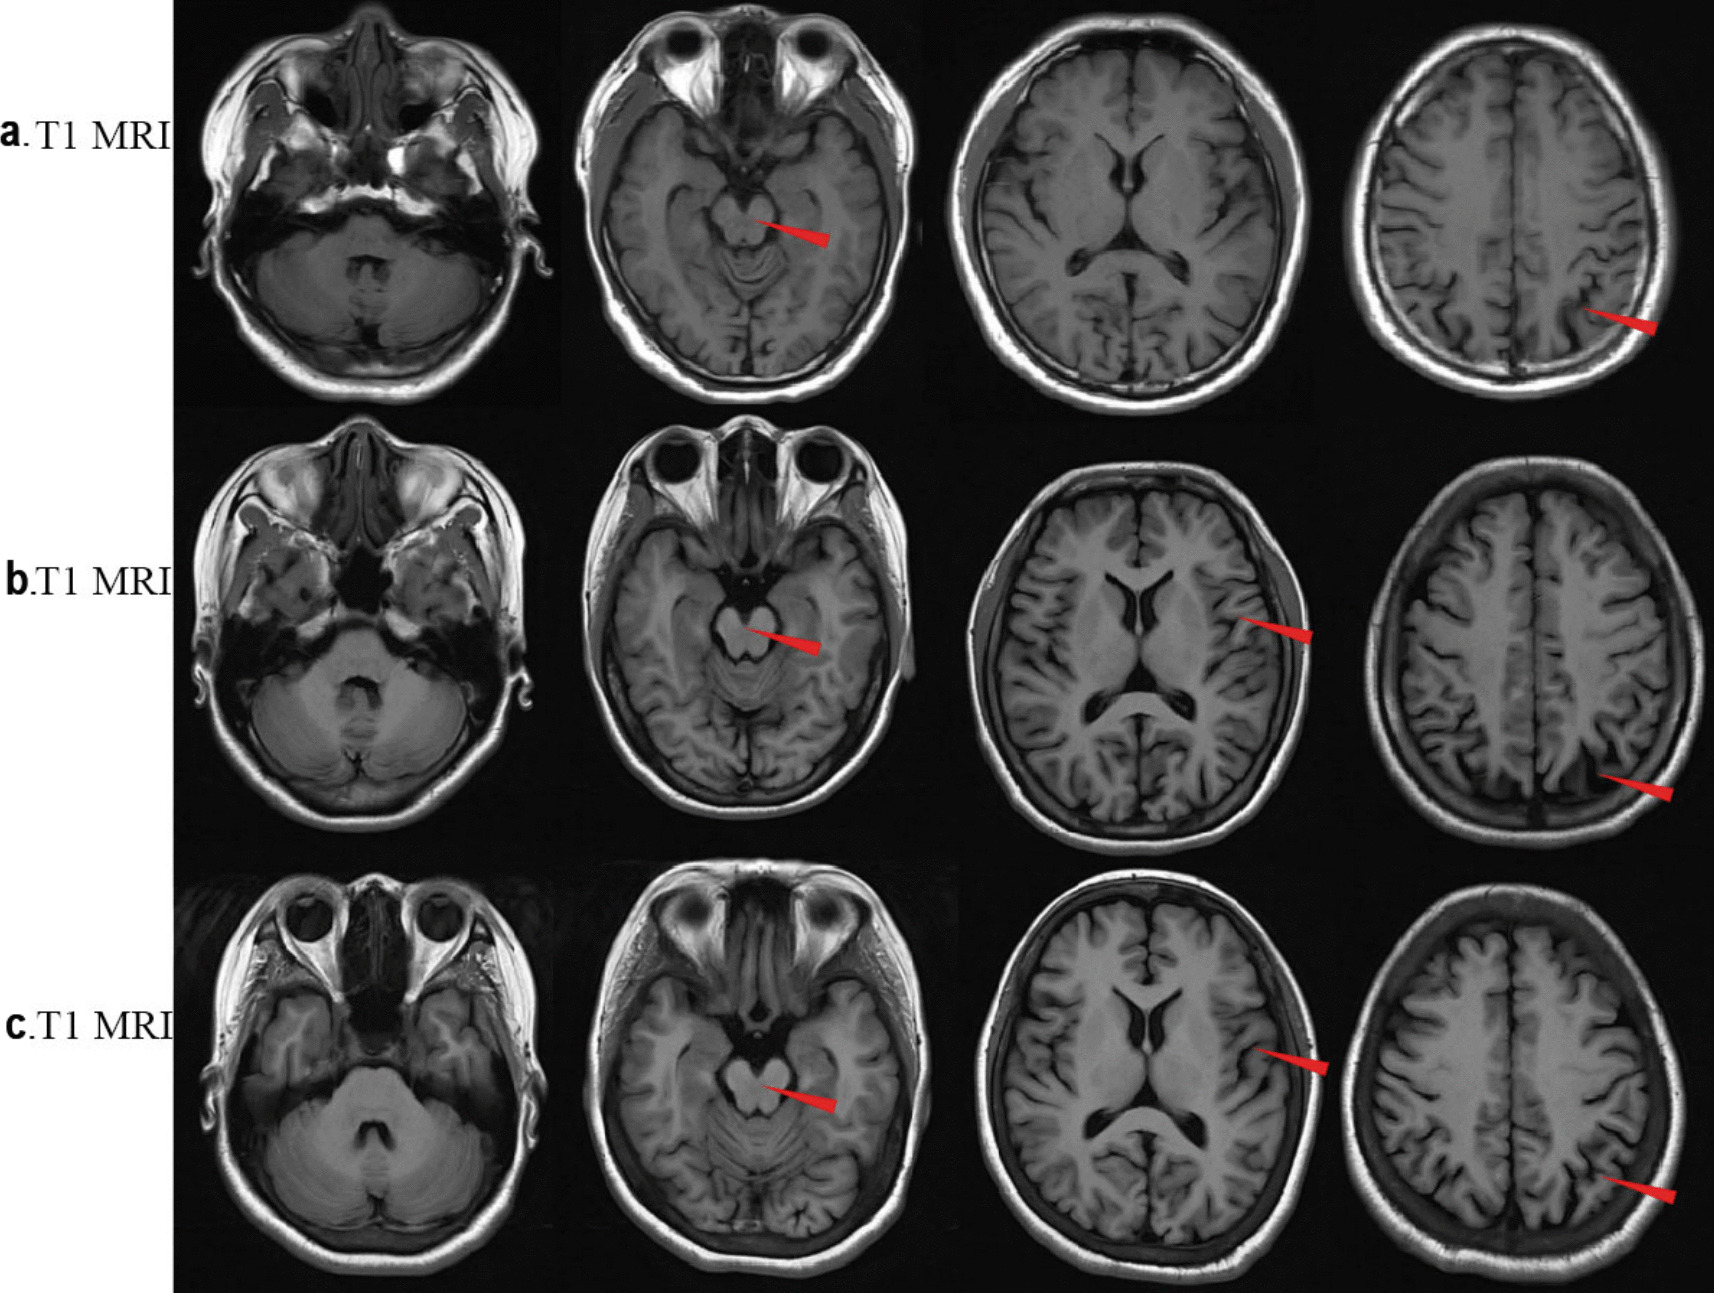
Axial T1 brain magnetic resonance imaging (MRI) of the patients both show brain atrophy. a. the reported patient. b. The second elder sister of the patient; c.The eldest sister of the patient
